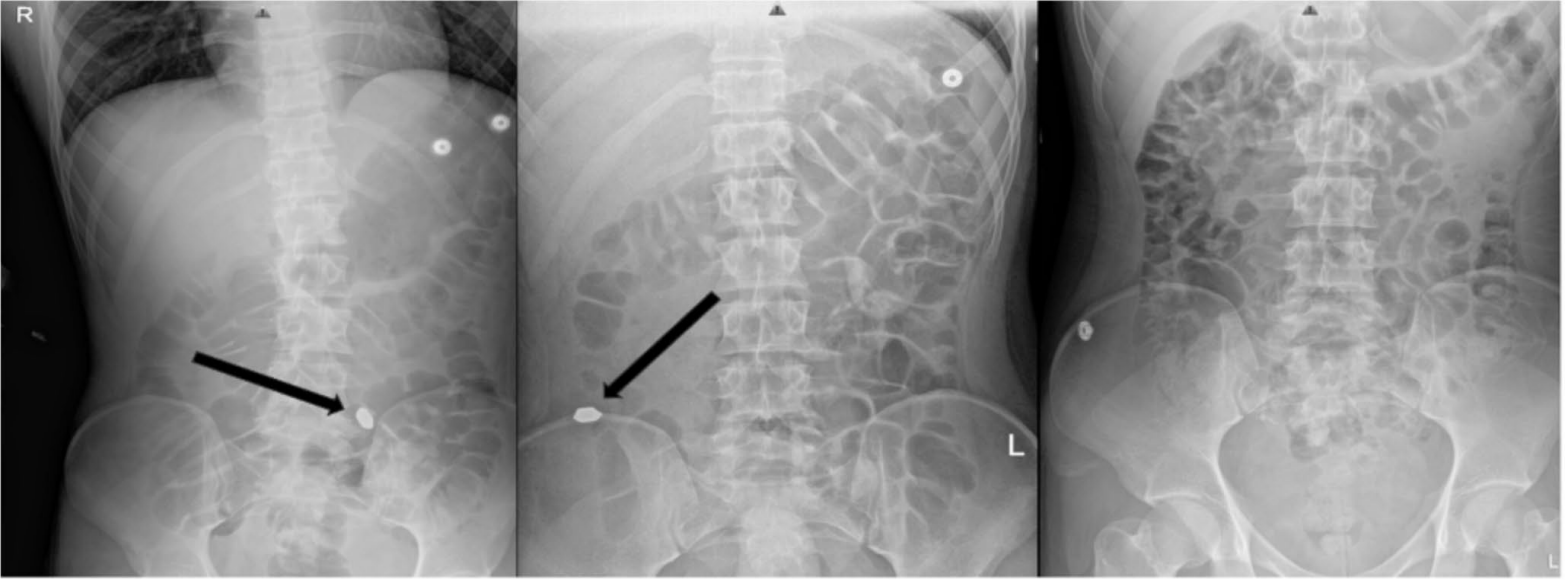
Abdominal X-rays of patient. Arrows: Radiopaque foreign body. Left 2a: Abdominal X-ray obtained on day of presentation, demonstrating radiopaque foreign body in left lower quadrant. Center 2b: Abdominal X-ray obtained on HD 2, demonstrating radiopaque foreign body in right lower quadrant. Right 2c: Abdominal X-ray obtained post-colonoscopy, demonstrating removal of radiopaque foreign body
